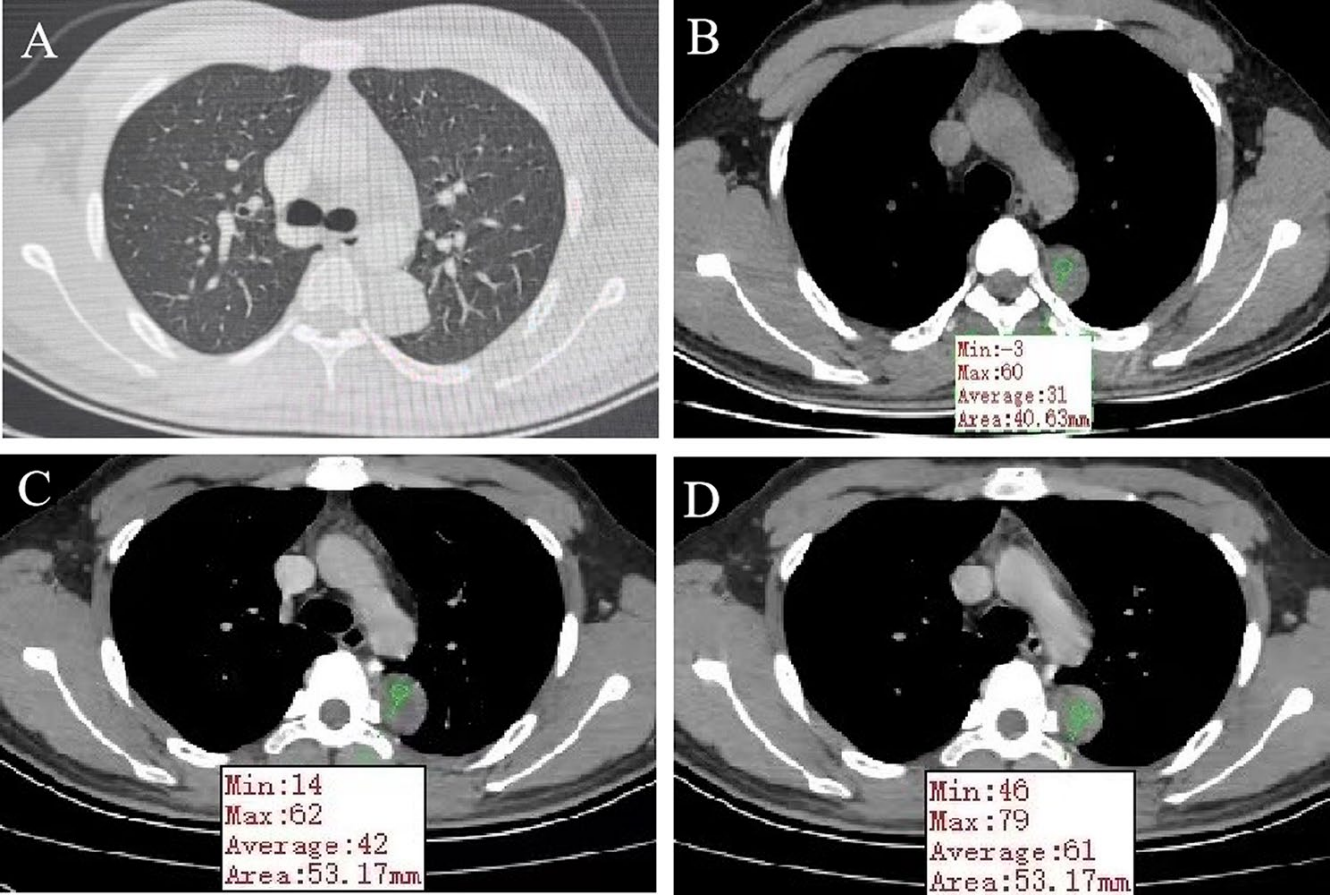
Computed tomography scans (A) revealed a mound-shaped soft tissue density shadow adjacent to the left posterior mediastinum at the fifth thoracic vertebra level, measuring approximately 4.0 × 2.8 × 2.1 cm, with well-defined borders and a broad base connecting to the inner left chest wall. Lung textures appeared normal, and bilateral pulmonary hila were not enlarged, with unobstructed airways bilaterally. Hilar lymph nodes and mediastinal lymph nodes were not enlarged. (B) Plain scan lesion CT value 31HU. (C) The CT value of arterial phase lesions was 42HU. (D) The CT value of the lesions in the venous phase was measured at 61 Hounsfield units (HU)
